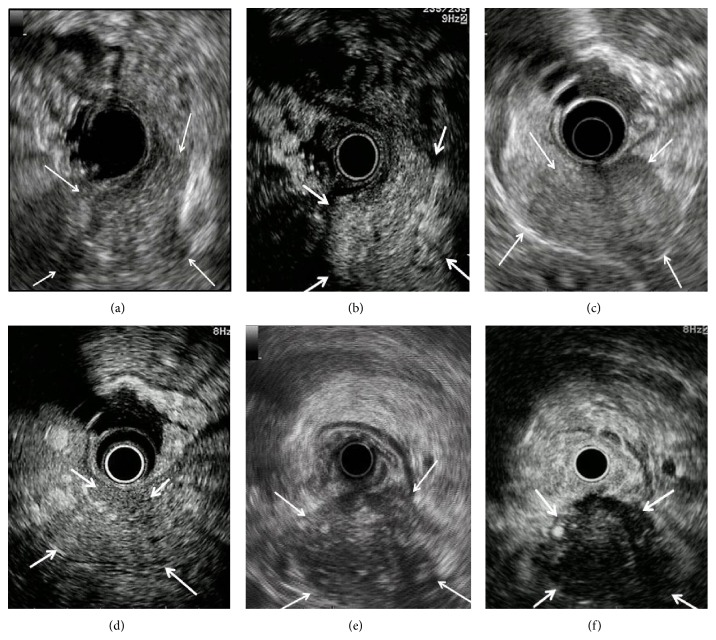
Evaluation of vascular pattern by contrast-enhanced endoscopic ultrasonography (CE-EUS). A representative case of tumors with hypervascular pattern: pancreatic lesion was detected as a low echoic lesion (arrow) with B-mode EUS (a). CE-EUS detected a pancreatic lesion with hyperintensity of enhancement (arrow) as compared to that of surrounding pancreatic tissue (b). A representative case of tumors with isovascular pattern: pancreatic lesion was detected as a low echoic lesion (arrow) with B-mode EUS (c). CE-EUS detected a pancreatic lesion with isointensity of enhancement (arrow) as compared to that of surrounding pancreatic tissue (d). A representative case of tumors with hypovascular pattern: pancreatic lesion was detected as a low echoic lesion (arrow) with B-mode EUS (e). CE-EUS detected a pancreatic lesion with hypo-intensity of enhancement (arrow) as compared to that of surrounding pancreatic tissue (f).
